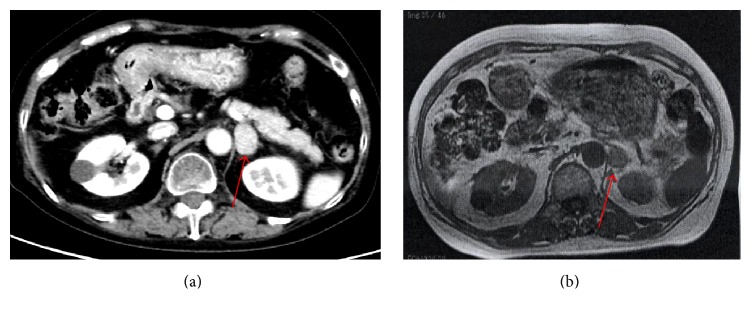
A 62-year-old woman with Cushing's syndrome: (a) computed tomography and (b) magnetic resonance imaging showed an adrenal tumor (29 × 22 mm, red arrow).
